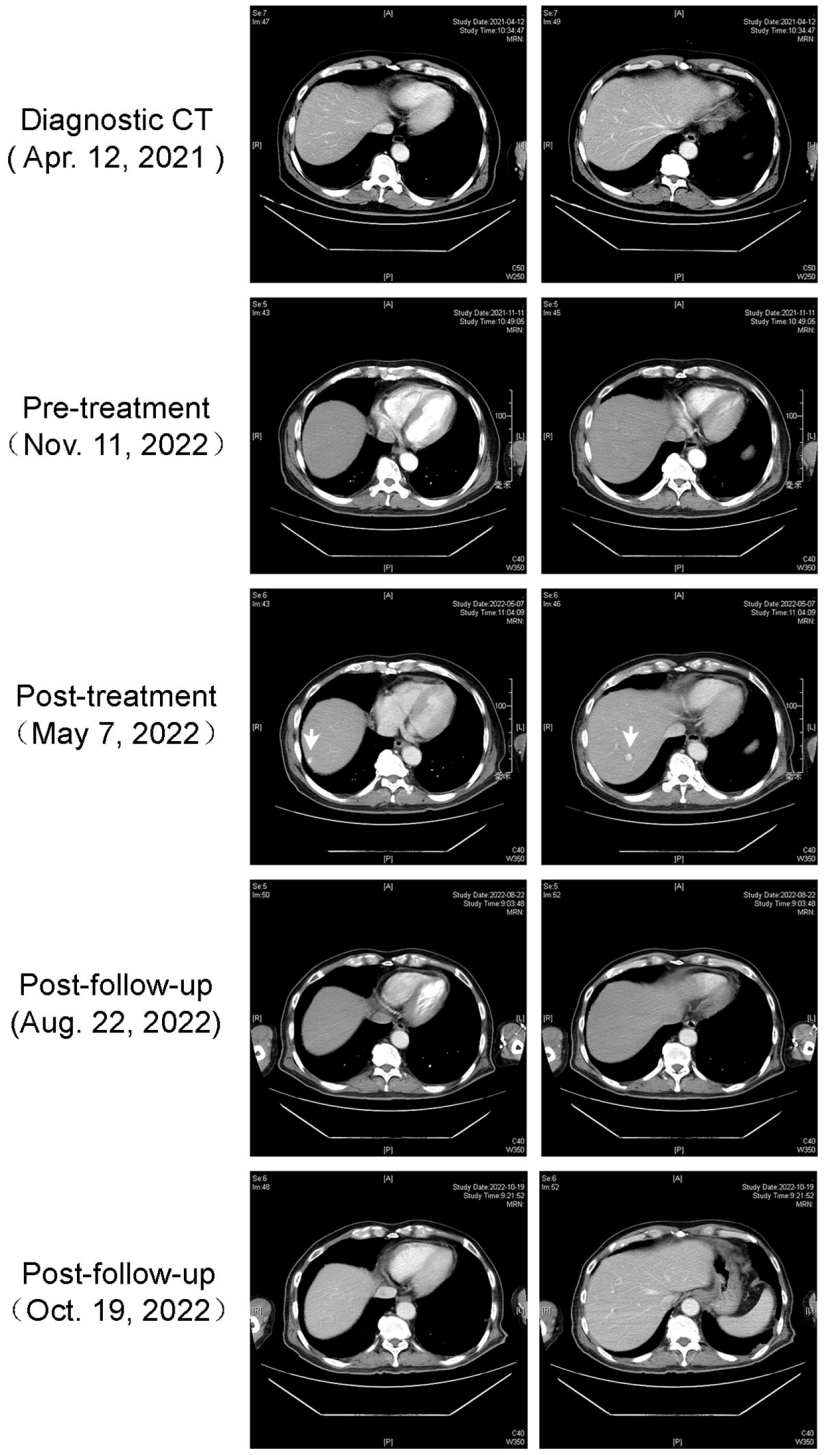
Case 2 hepatic cavernous hemangioma imaging. Enhanced CT visualization of hepatic cavernous hemangioma in the second case.
